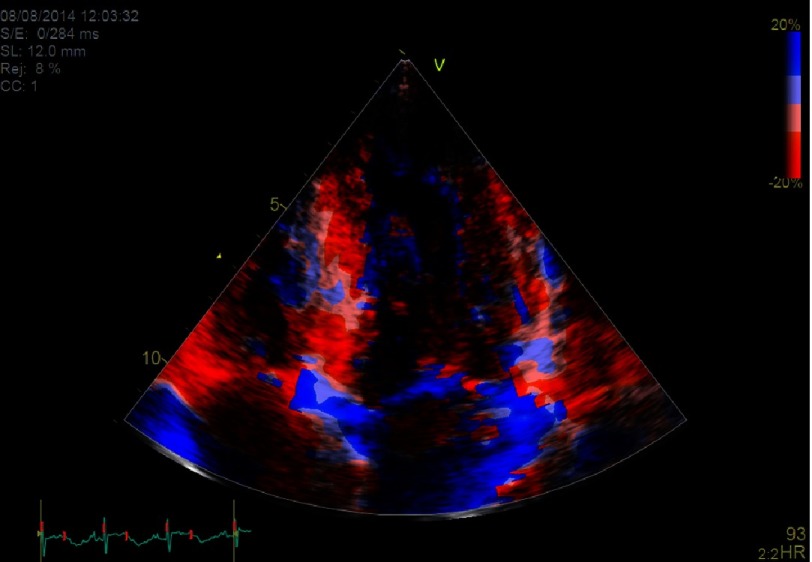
A patient with a sarcomere mutation positive HCM evaluated with color-coded Doppler Tissue Imaging. Different color-codes correspond to different deformation within the hypertrophied inferoseptum. Heterogeneity can be noted throughout the septum, with no deformation in parts of the mid-septum (*light red and blue color*).
